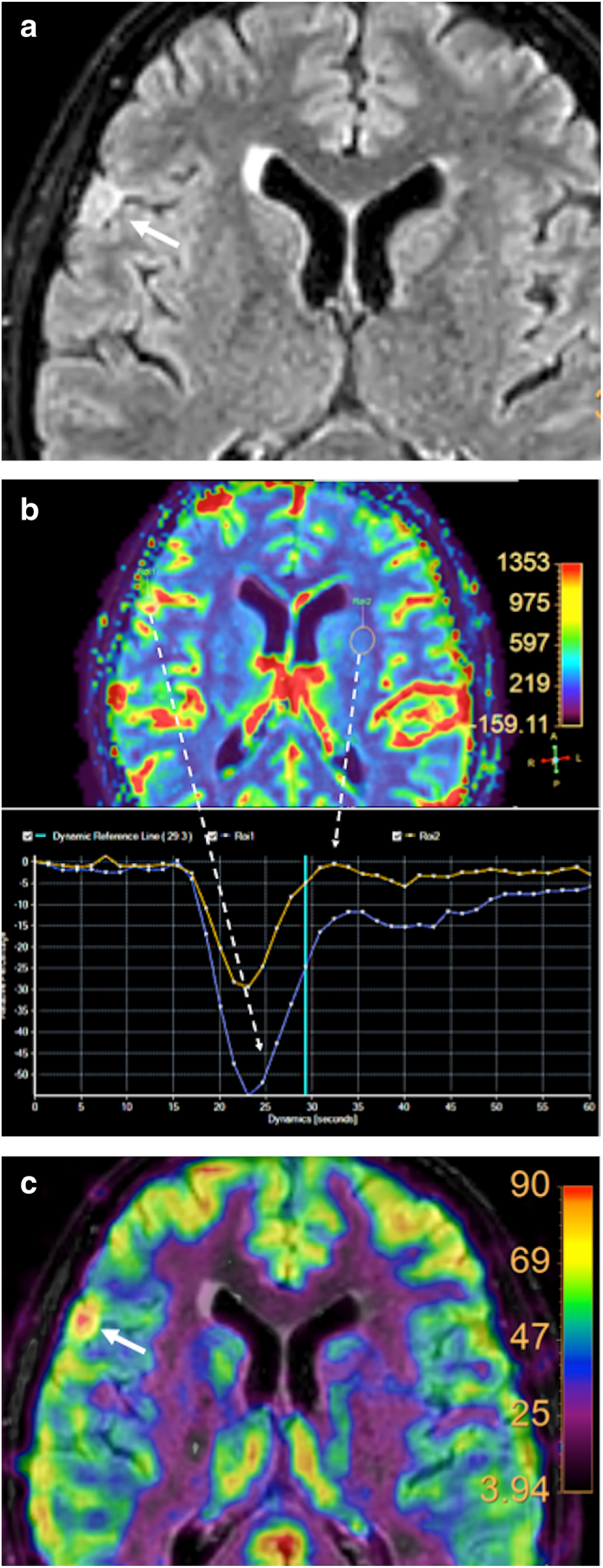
Brain perfusion in a 55-year-old woman with a meningioma. a An axial fluid attenuation inversion recovery (FLAIR) image of the brain demonstrated a hyperintense extra-axial insular lesion (white arrow). Both (b) dynamic susceptibility contrast (DSC)-MRI and (c) arterial spin labelling (ASL) parametric maps demonstrated and increased perfusion in the mass. Comparison to normal brain parenchyma evdenced a higher decrease in MR signal intensity curves during the first-pass of the contrast bolus (b, blue curve)
